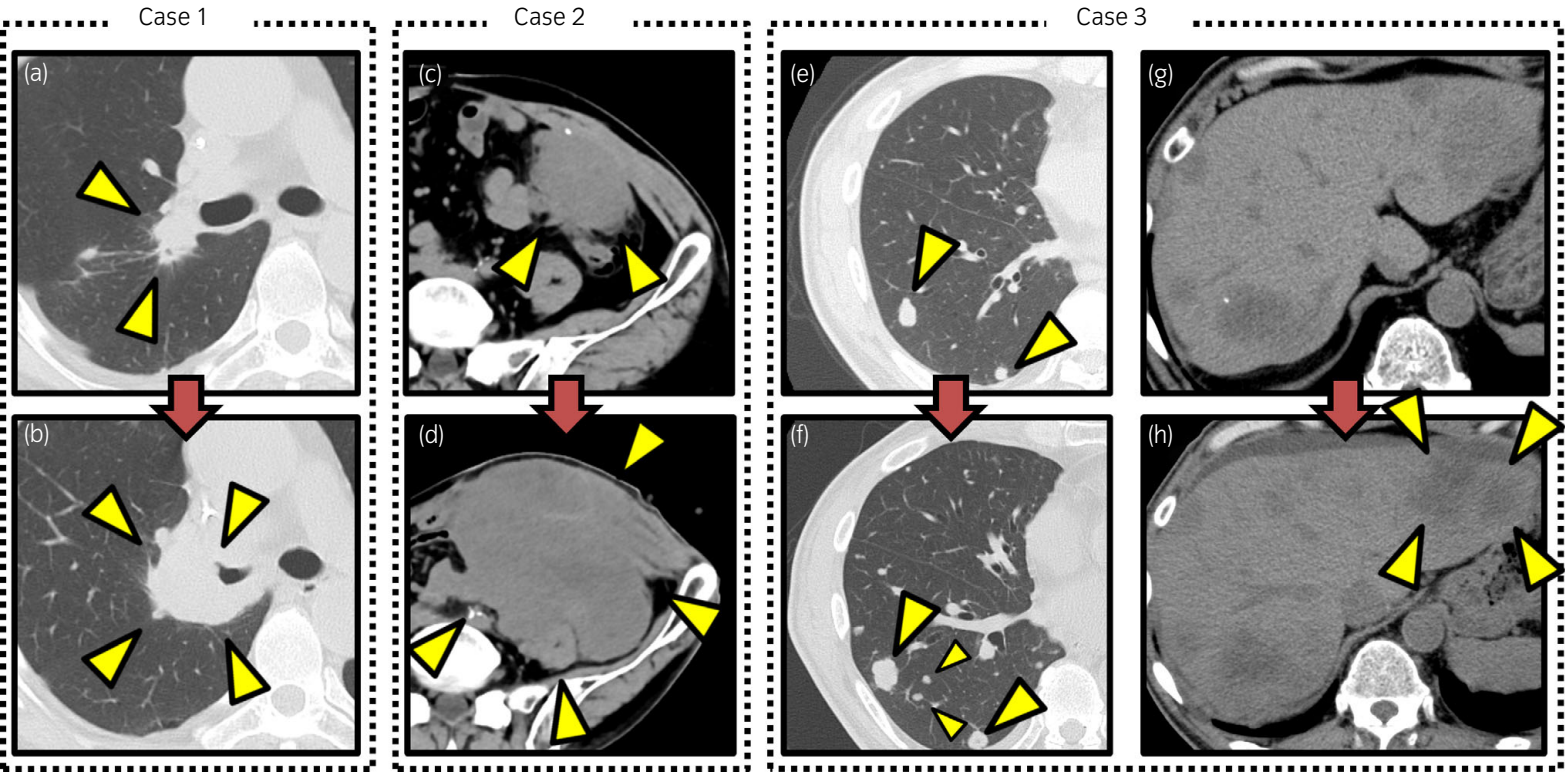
CT images before and after pembrolizumab rechallenge. (a, b) CT images in Case 1. The middle lobe metastasis in the right lung developed before pembrolizumab rechallenge (a). After five doses, the lung metastasis progressed, and the right main bronchus was compressed (b). (c, d) CT images in Case 2. Peritoneal metastasis developed before the pembrolizumab rechallenge. PD was observed immediately after three doses of pembrolizumab rechallenge (d). (e, h) CT images in Case 3. New multiple lungs and liver metastases recurred before the pembrolizumab rechallenge (e, f). After two doses of pembrolizumab rechallenge, rapid progression was observed (g, h).
